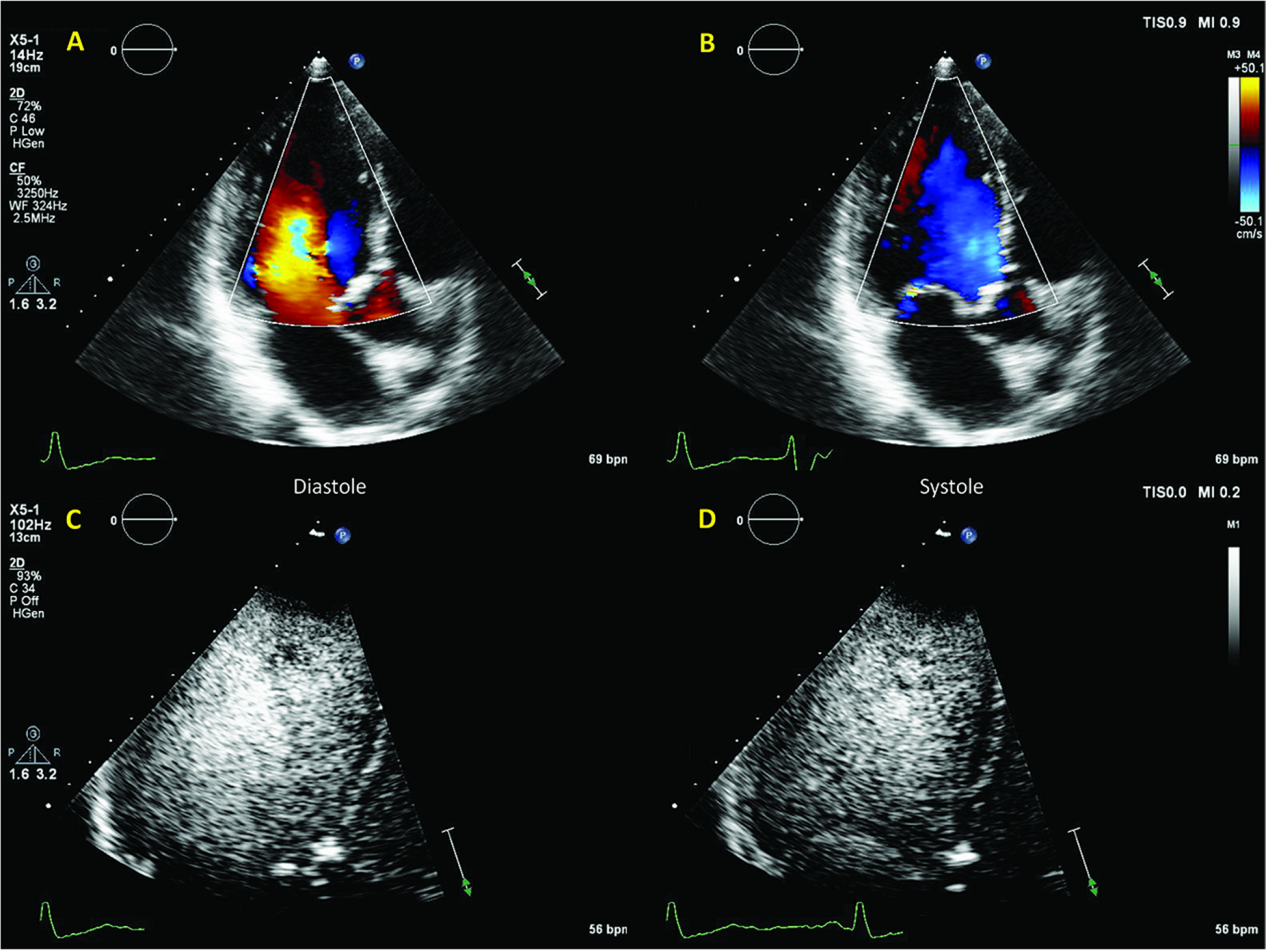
Patient 4. A, B: color Doppler of the LV. Dilated LV and low-flow. The AI jet is not clear; C, D: clinical contrast imaging not diagnostic in spite of good image quality
